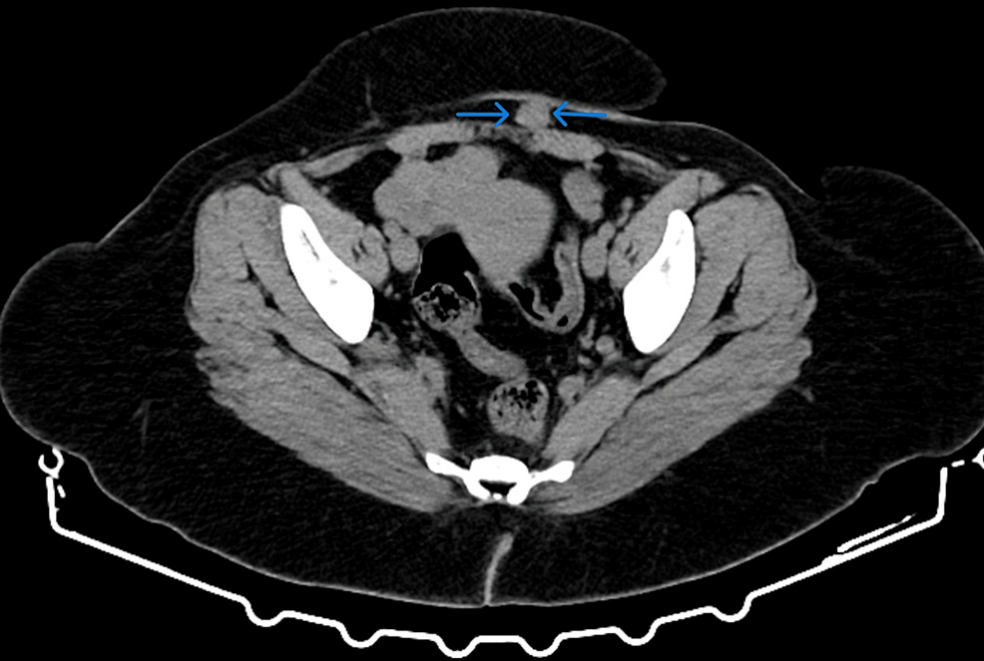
Computed tomography imaging of abdominal wall endometriosis A round lesion (blue arrows), isodense to the muscle tissue, is observed within the subcutaneous tissue and adjacent to the rectus abdominis muscle fascia, exhibiting marked and homogeneous enhancement
